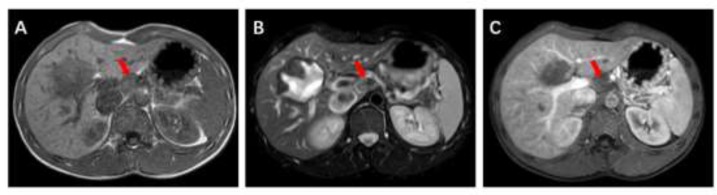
MRI scan. A (T1), B (T2) Sequence and C (enhanced scan) showed enlarged lymph node in hepatogastric space with slightly longer T2 signal.
